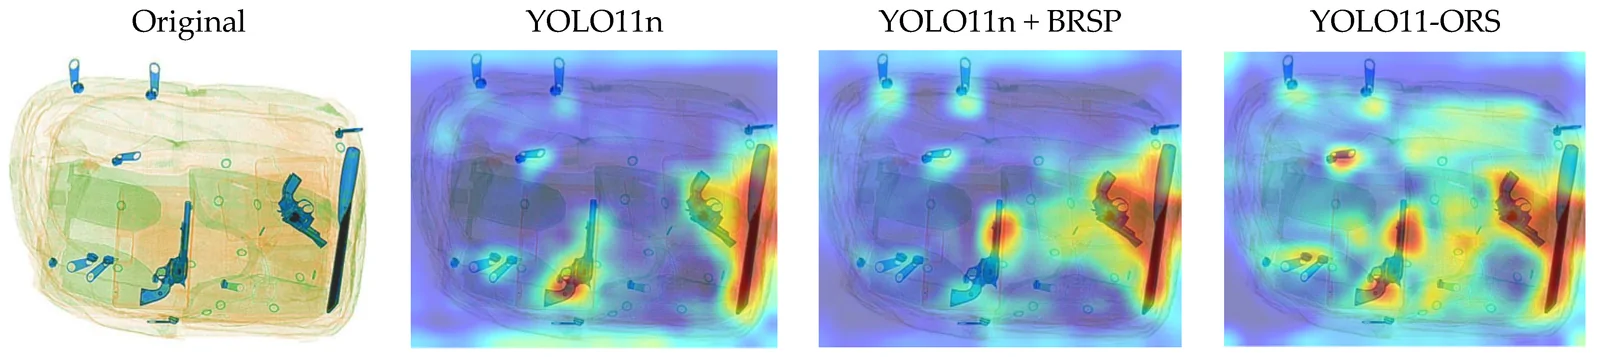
Visualization of deep feature responses for background redundancy suppression on a representative cluttered-background sample from SIXray. From left to right: original X-ray image, YOLO11n, YOLO11n + BRSP, and YOLO11-ORS.
